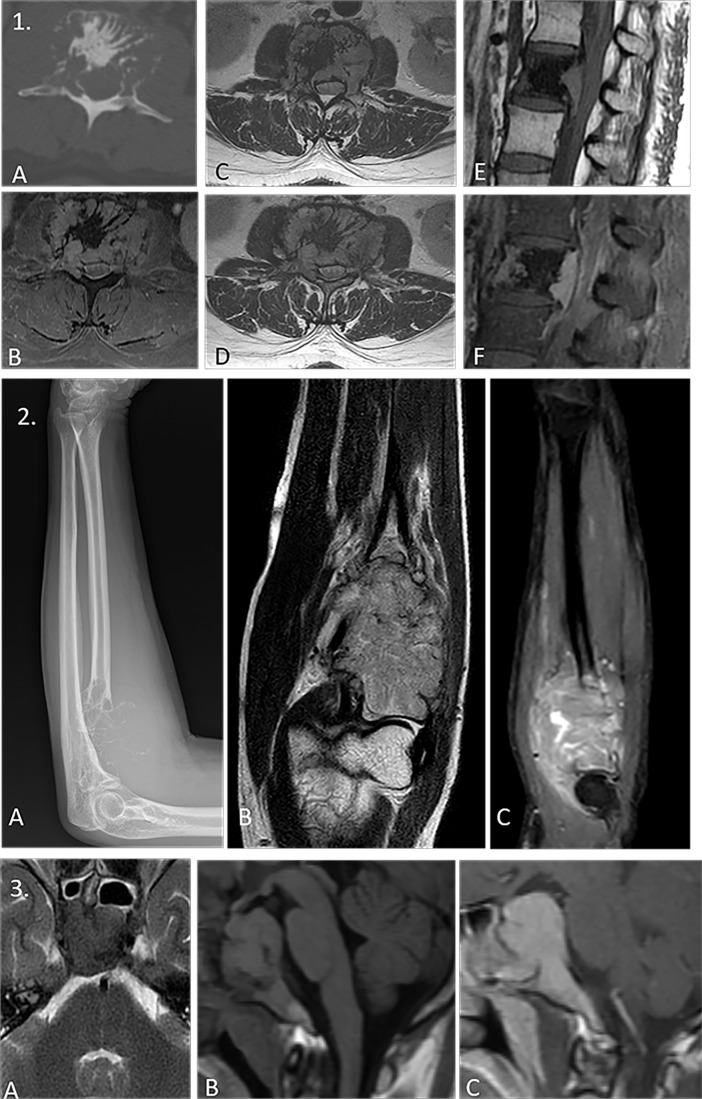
(1) Panel of images demonstrating the classic “mini-brain” appearance in a case of plasmacytoma on therapy with multiple hypointense struts seen emanating from the L2 vertebral body lesion seen on axial computed tomography (CT) [image (A)], axial STIR [image (B)], axial T1WI [image (C)], and axial T2WI [image (D)]. Note the central sclerosis representing post-therapy changes. The non-sclerotic component shows intense post-contrast enhancement with retropulsion as seen in pre-contrast sagittal T1W [image (E)] and post-contrast sagittal images [image (F)]. (2) Solitary plasmacytoma mimicking a soap-bubble lesion. A large expansile multilobulated lesion is seen involving the proximal radius on the radiograph [image (A)]. Coronal T2WI MRI image shows an intermediate T2 signal intensity lesion [image (B)] which shows post-contrast enhancement [image (C)]. Differentials of such a radiologic appearance include the giant cell tumor and blowout metastases. (3) Expansile lesion involving the clivus with T2 iso- to hypointense signal intensity [image (A)], T1 isointense signal intensity [image B], and intense post-contrast enhancement [image (C)]. The lack of T2 hyperintensity makes both chordoma and chondrosarcoma (lesions classically affecting the clivus) highly unlikely. Endonasal sampling revealed features of plasma cell neoplasm.
